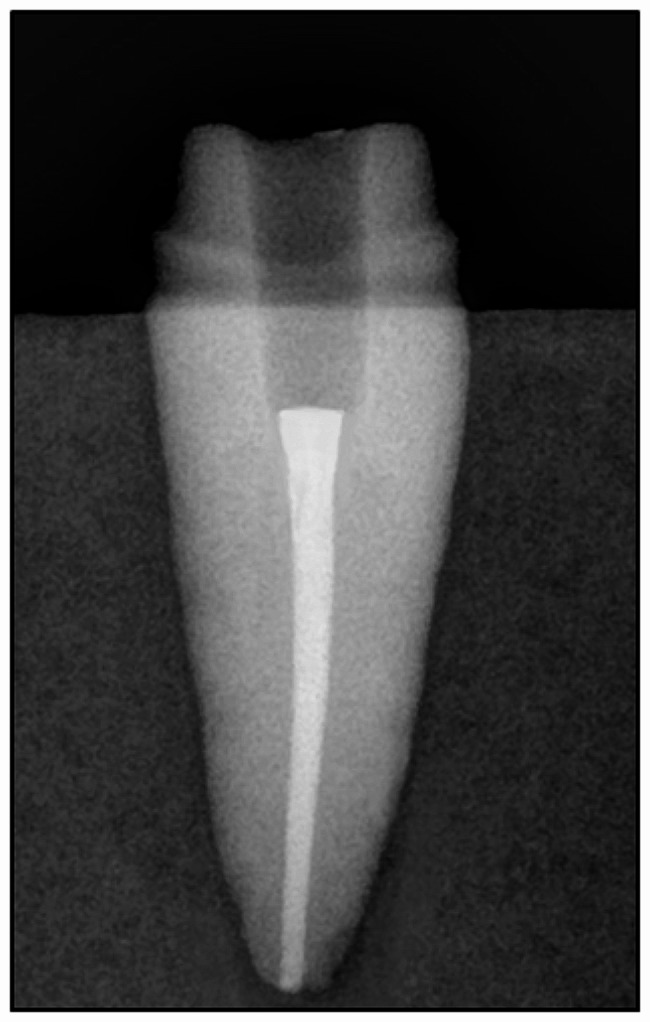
X-ray of tooth intra-radicular preparation for endocrown
